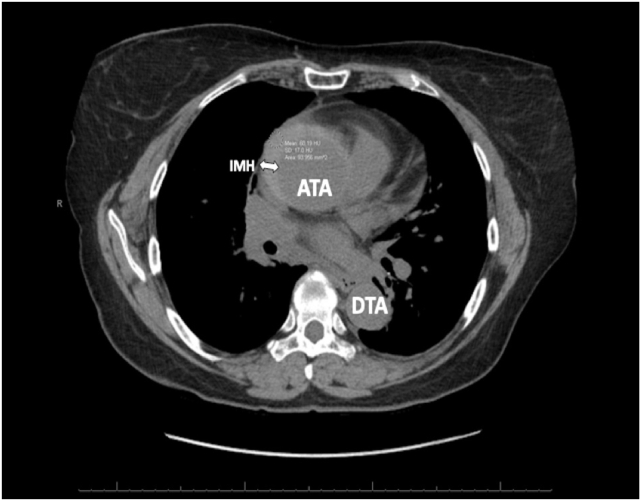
Nonenhanced CT scan, axial display at the level of the proximal ascending thoracic aorta, demonstrates a crescentic, high-attenuation (relative to the aortic lumen) region of aortic wall thickening that measures 60 Hounsfield units and strongly suggests an IMH (*arrow*). *ATA*, Ascending thoracic aorta; *DTA*, descending thoracic aorta.
